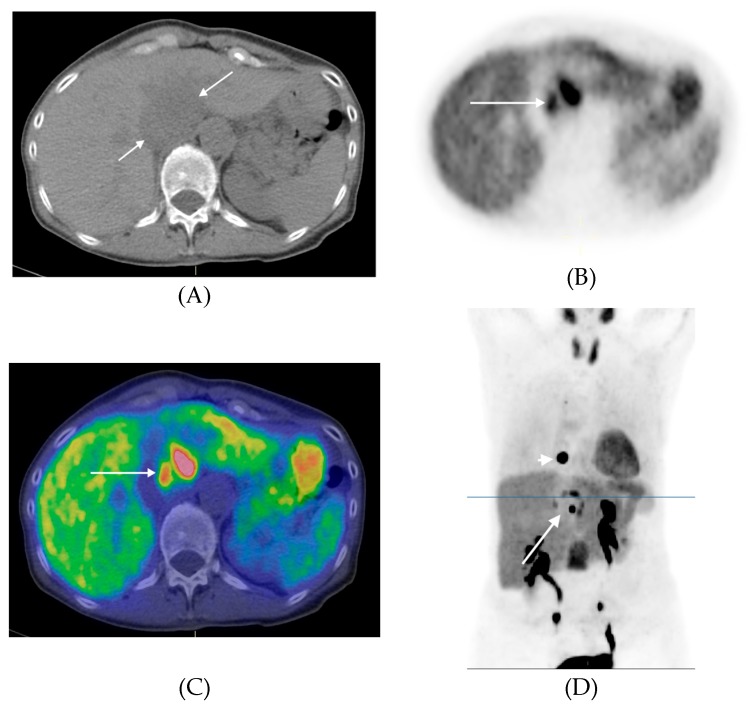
^11^C-HED-PET/CT of patient #24 (Table 4) with a retroperitoneal paraganglioma in front of the descending aorta with high heterogenous tracer uptake (long arrows) and a metastasis in the transverse process of the thoracic vertebra 10 shown in D (short arrow). In the coronal PET image an additional vertebral metastasis in the first lumbar vertebra is seen projected between the kidneys. (A) Transversal CT, (B) Transversal PET, (C) Transversal PET/CT fusion, (D) Coronal PET (Maximum Intensity Projection). The level of the transversal images are indicated in the coronal PET image (line).
